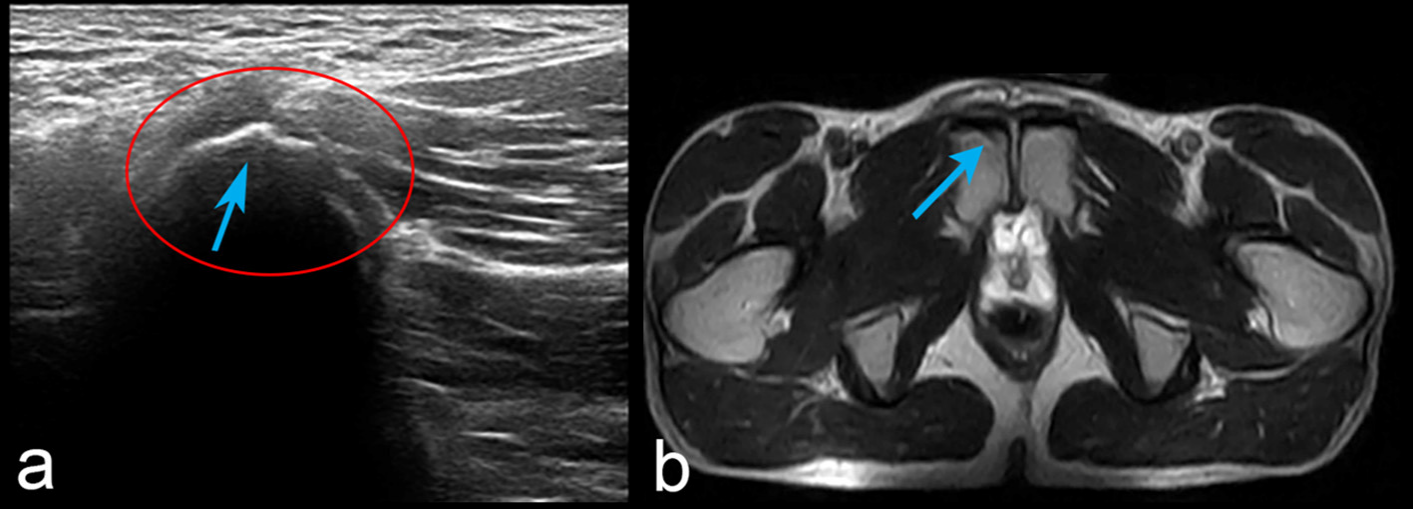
Ultrasound (a) and MRI investigation on the axial plane (b). Both images highlight a reduction of the phlogistic phenomenon (blue arrow). The cortical profile irregularity, however, remains.
